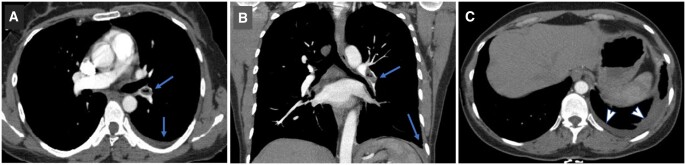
CT images at initial diagnosis, filling defect and pleural fluid are indicated by arrows, on axial plane (A) and on coronal plane (B). The atelectasis is indicated by arrowheads on axial plane (C).
